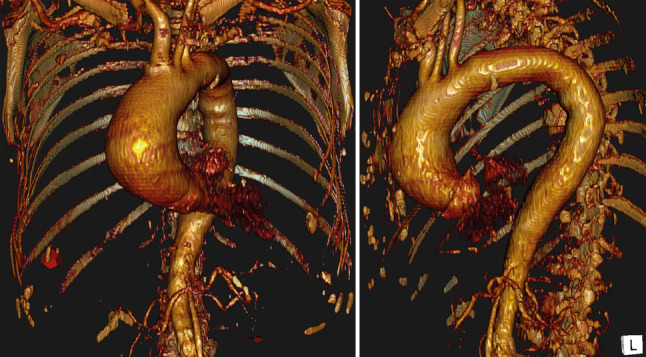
Preoperative computed tomography reconstruction of an 81-year-old female with ascending aorta dilatation
